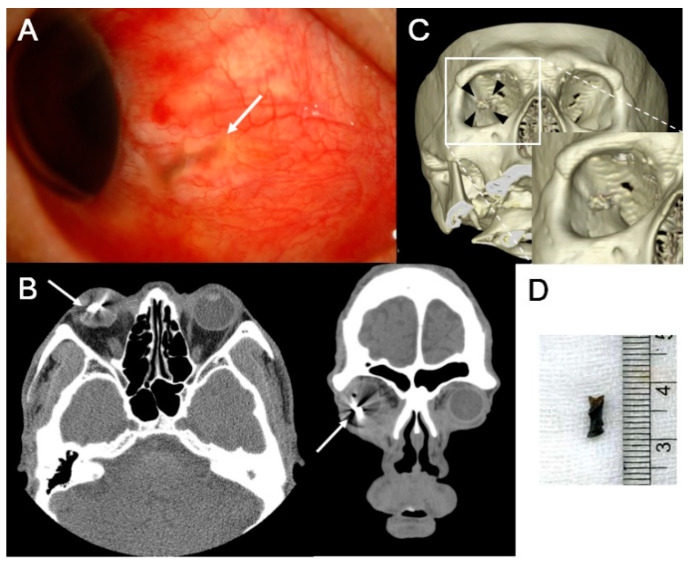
Anterior segment photograph and CT scan of a 54-year-old male patient struck by a metallic foreign body in his right eye during work. (A) A laceration site in the nasal sclera (*long white arrow*) is observed as an inlet of the intraocular foreign body (IOFB). (B) Axial and coronal CT scan of the patient shows the hyperdense foreign body (*long white arrows*) in the vitreous cavity and intraocular air. (C) On the 3D reconstructed image, the shape of the IOFB (*black arrowheads*) is better documented, which is more remarkable on magnified images (*white box*). (D) The length of the removed metallic foreign body is 7.5 mm.
